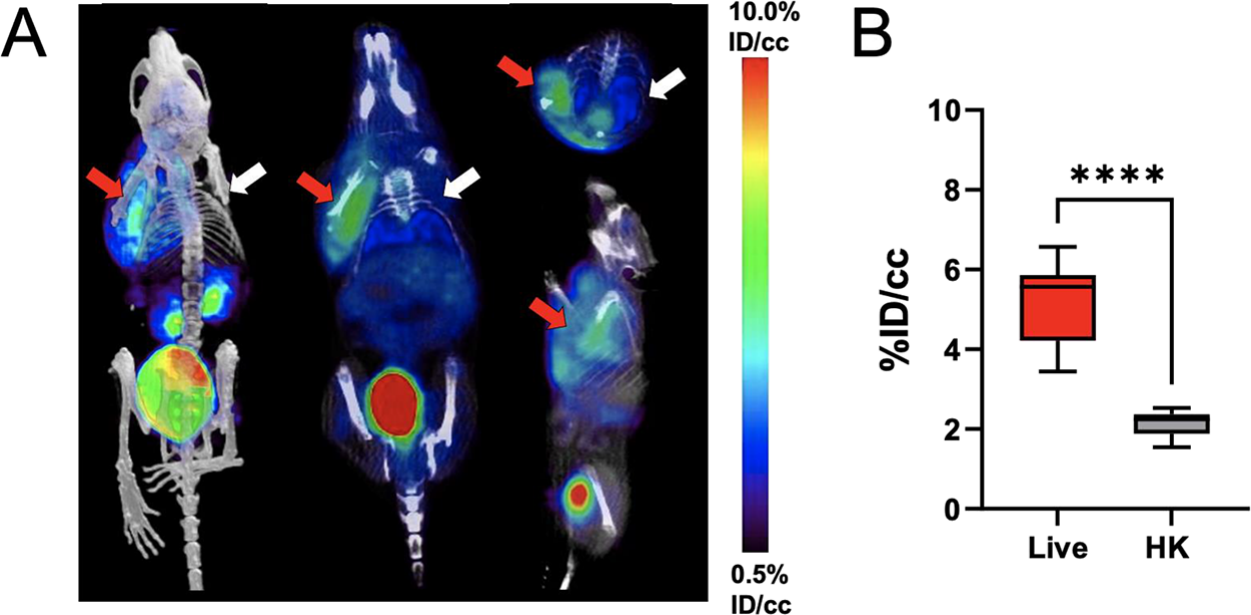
μPET/CT imaging analysis of d-[^18^F]-CF_3_-ala in *E. coli*-infected
